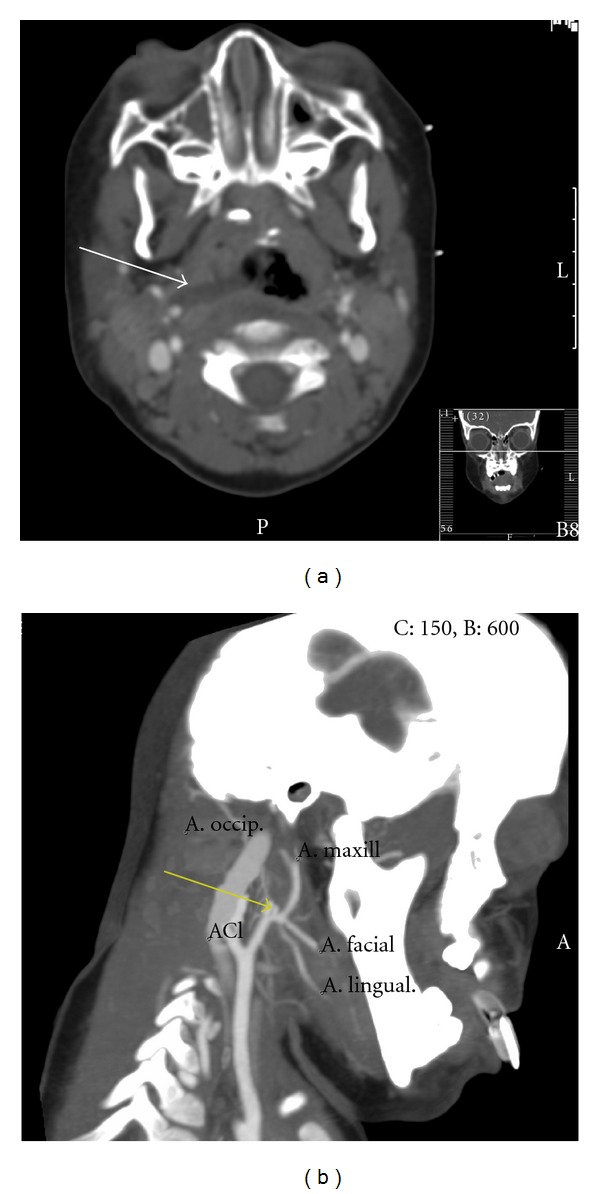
CT angiography of the head and neck showing a hematoma shaped like the head of the toothbrush in the right nasopharynx (white arrow) and the aneurysmatic dilatation of the origin of the internal maxillary artery (yellow arrow).
